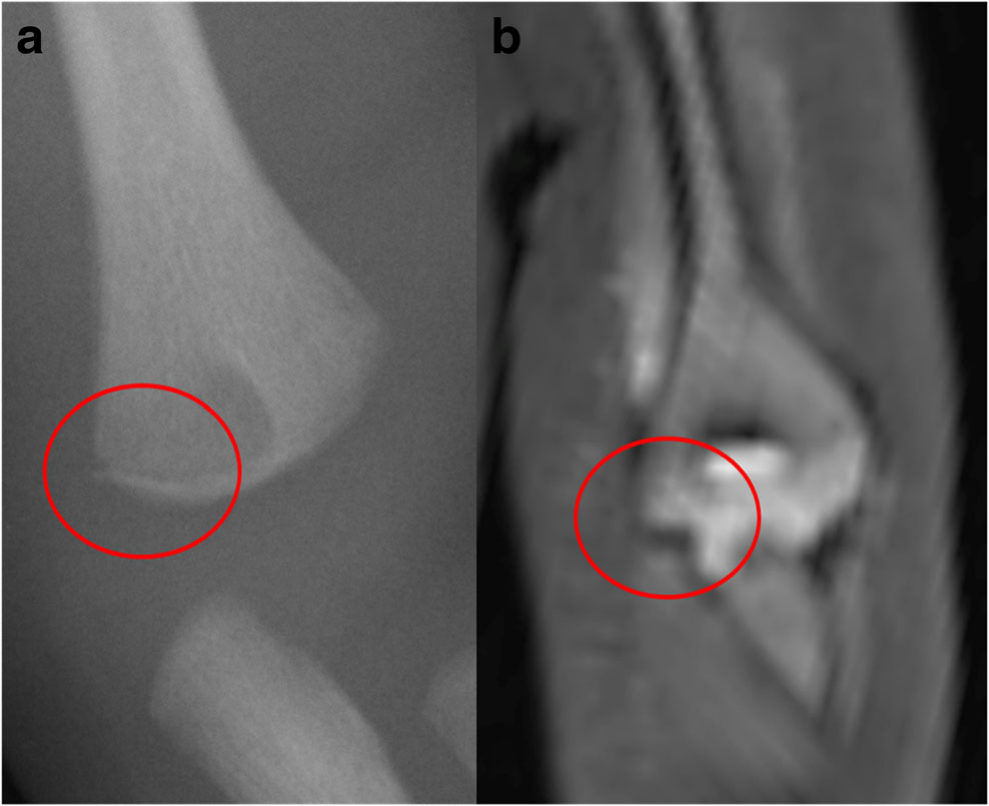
Fracture detection on PMMR. Long bone fractures are still probably best imaged using conventional radiographs, as these demonstrate long bone fractures adequately and some subtle fractures may be missed on PMMR, such as this corner metaphyseal fracture of the left distal humerus in a 5 month old child. It was identified on conventional radiography (a) but deemed too subtle to be identified on PMMR (b). Reproduced with permission from [16]
